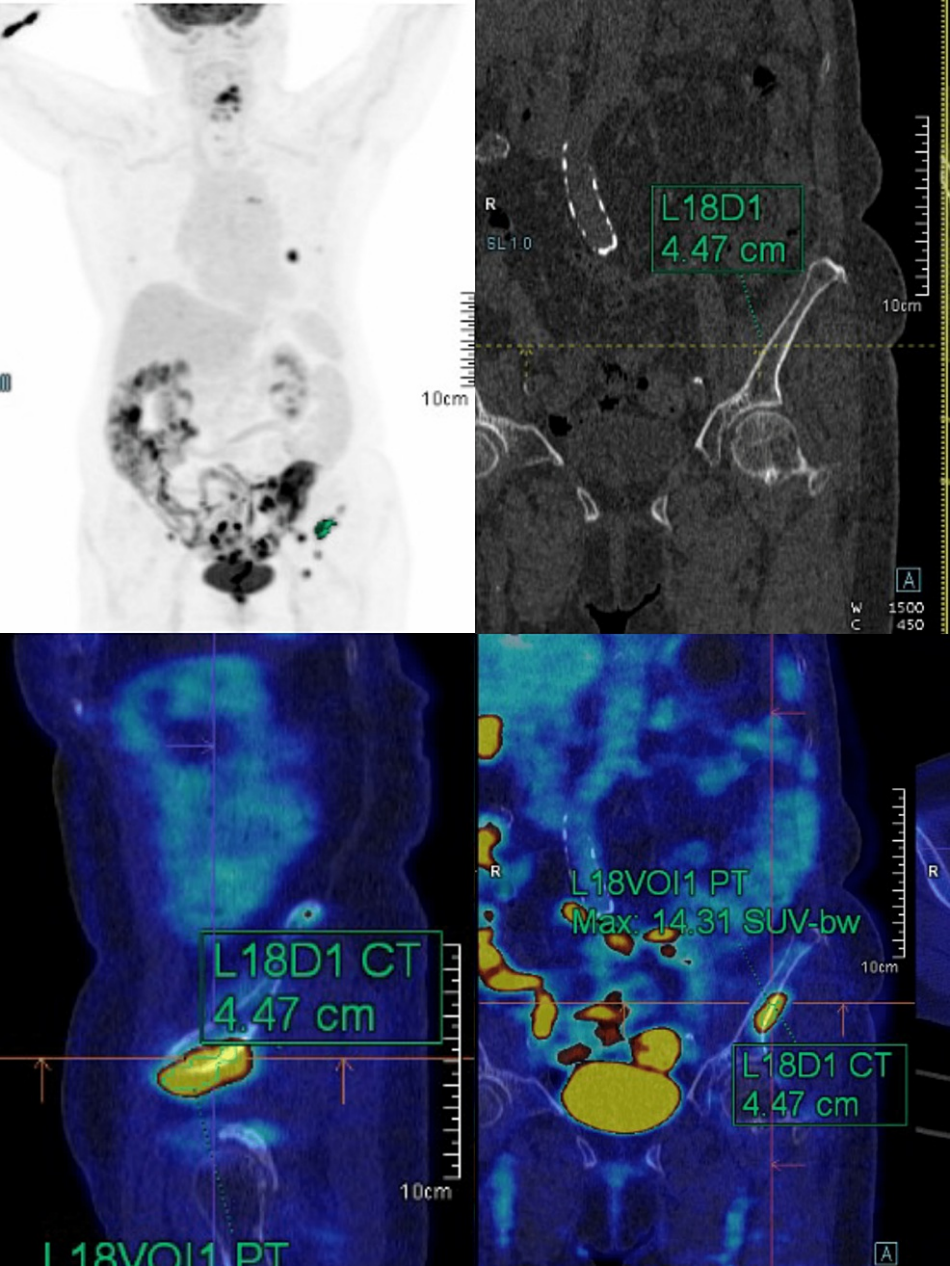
Whole-body PET-CT Sixty minutes after the intravenous injection of 18F-FDG, a non-contrast CT was obtained from the base of the skull to the upper thigh for attenuation correction and anatomic localization of radiotracer activity. Emission PET and post-contrast CT scans were obtained from the same anatomical regions. Images were reconstructed and used to identify a hypermetabolic lung nodule (1.91 x 1.6 cm/SUVmax 15.61), shotty aortopulmonary lymph nodes (0.57 cm/SUVmax 4.55 and 0.67 cm/SUVmax 6.17), and hypermetabolic skeletal lesions on the left ilium: (4.47 cm/SUVmax 14.31). These findings suggested lung, nodal, and bone metastases. PET-CT, positron emission tomography-computed tomography; FDG, fluorodeoxyglucose.
